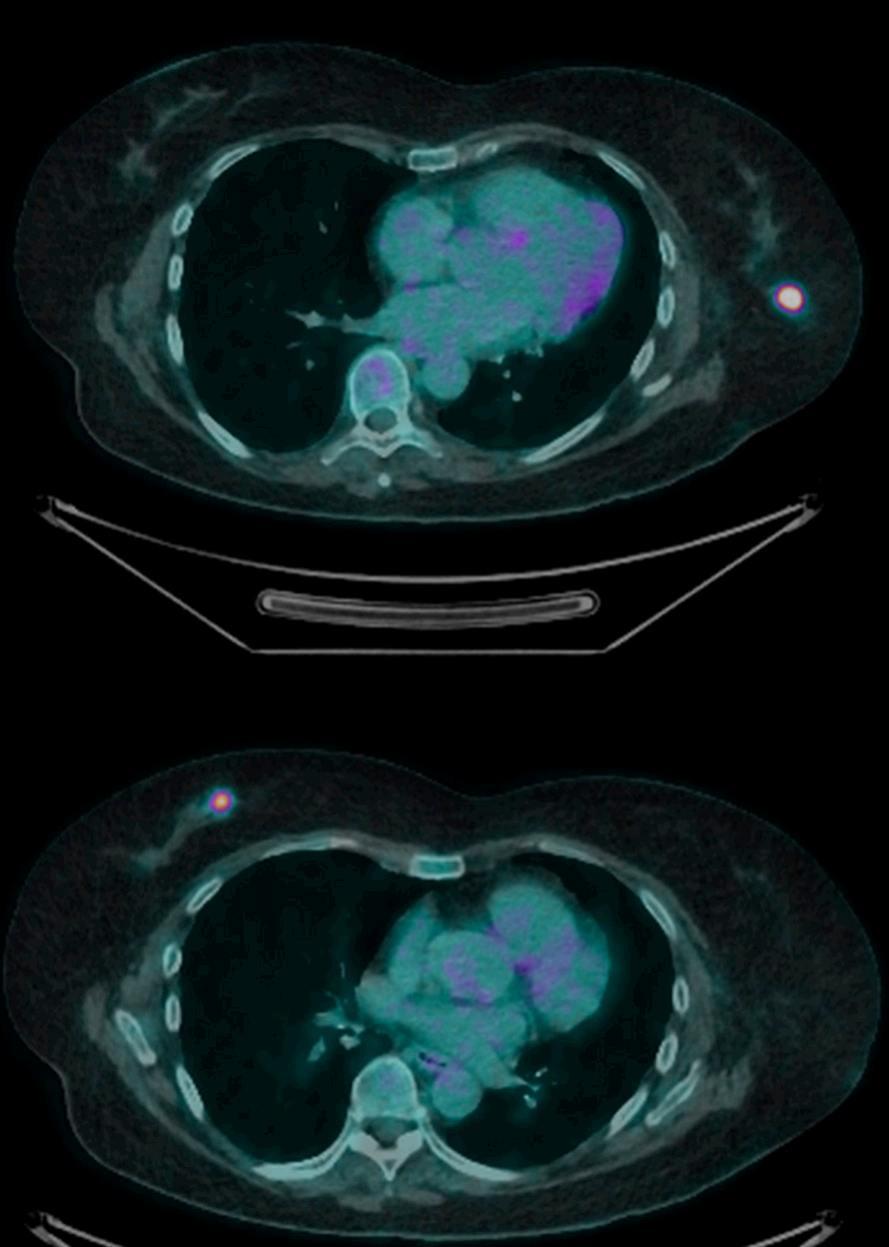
FDG PET CT with avid right-sided breast lesion (7a) and left-sided intramammary lymph node (7b). FDG, fludeoxyglucose; PET, positron emission tomography.
